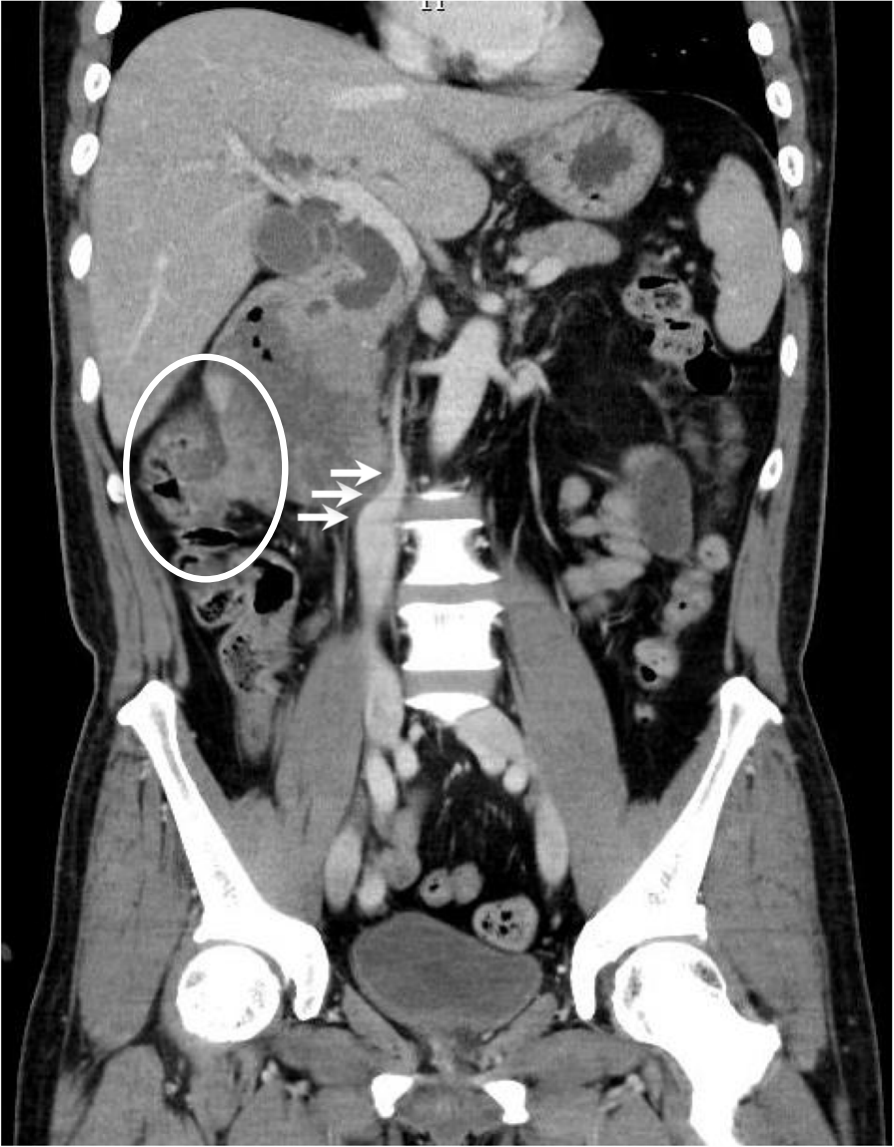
Computed tomography scan showed a greater than 10 cm tumor with heterogeneous enhancement. The central portion contained air suggesting necrosis and infection. No obvious metastases were seen in other abdominal viscera lung fields, and intraperitoneal lymph nodes were not enlarged. Arrows point to the compressed inferior vena cava. The circle shows infiltration to the ascending colon
